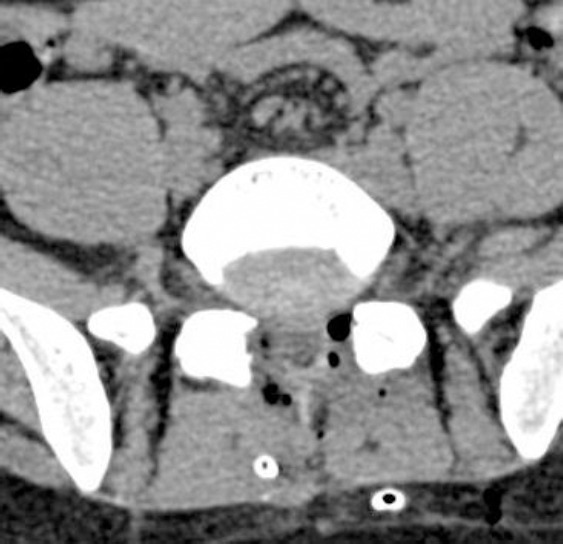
The appearance of the air in laminectomy field and the under-fascia part of the lumbar vacuum suction device on postoperative lumbar CT*.
